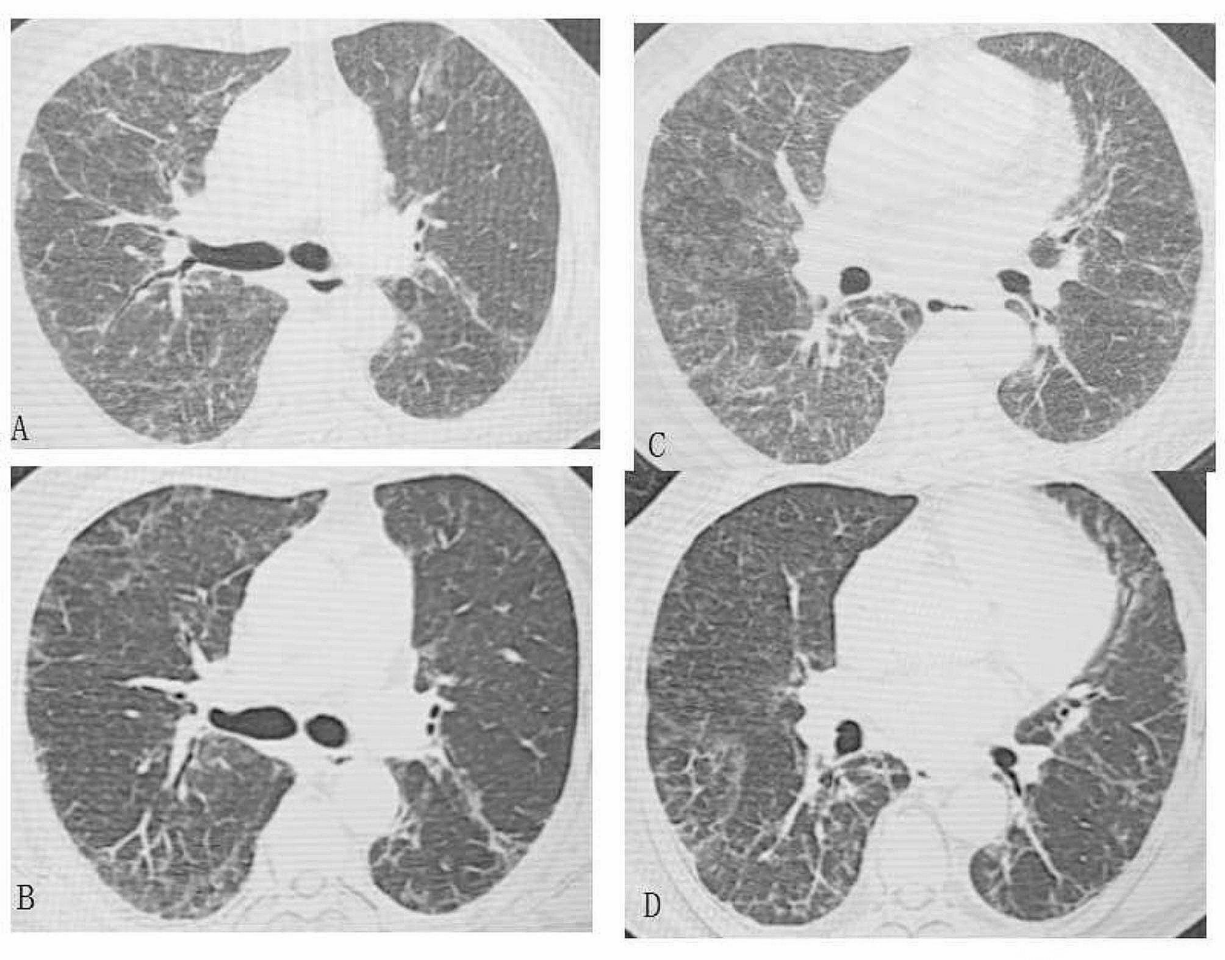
The CT scan after antibiotic administration (B, D) shows the diffuse infiltration was partially absorbed. The lung field was clearer than before. Chest CT scan before the antibiotics is showed in A and C
